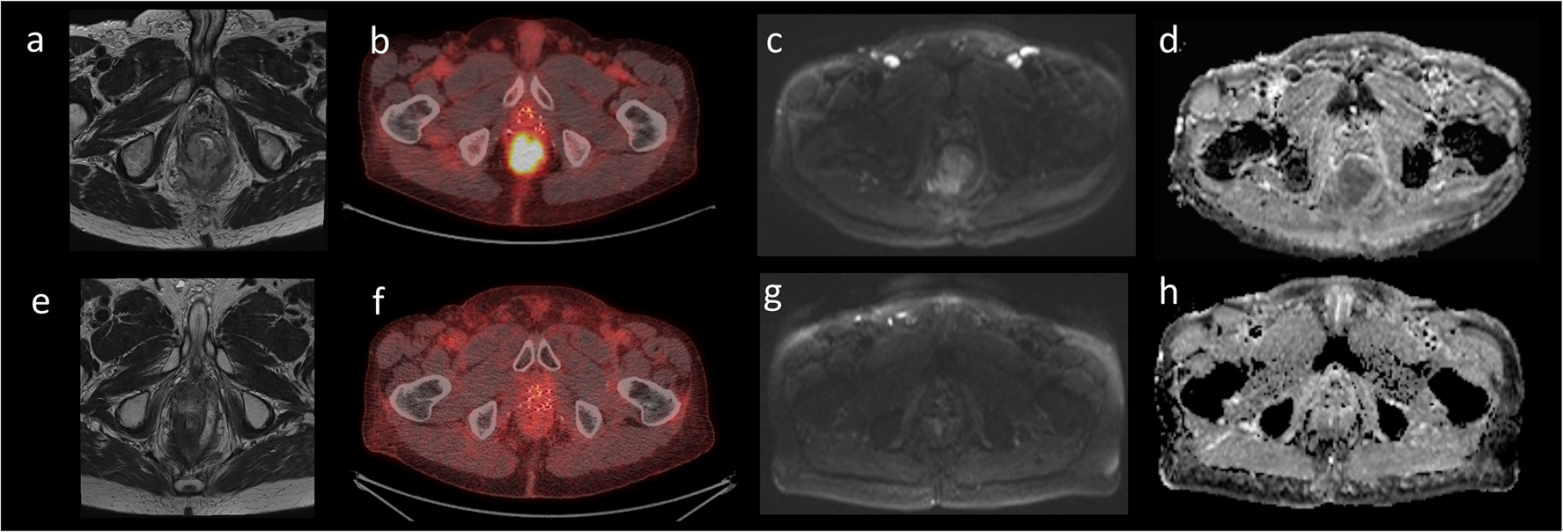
71-year-old male, brachytherapy seeds within the prostate. Axial images. MRI response assessment: partial, PET-CT response assessment: complete, combined response assessment: complete. a Pre-treatment T2W MRI demonstrates an almost annular locally advanced tumour centred at the anorectal junction extending into the perineum and rectum. b Pre-treatment PET-CT demonstrates a large metabolically active mass involving the anal canal and lower rectum; bilateral groin lymph nodes demonstrate moderate uptake and are likely involved. c DWI demonstrates diffusion restriction at the site of the tumour as well as inguinal node involvement (high signal). d The ADC confirms true restriction (low signal). e Post-treatment T2W MRI demonstrates reduced tumour volume which is largely fibrotic. Focal bowel wall oedema was present which may represent residual tumour or post-treatment inflammatory changes. No residual nodes are demonstrated. f Post-treatment PET-CT demonstrates no residual FDG avid disease at the site of the previous tumour and nodes. g The DWI sequence does not demonstrate restriction. h The lack of diffusion restriction is confirmed by the ADC map. Overall, due to the lack of residual FDG avid disease, the patient was considered as a complete responder. The focal bowel wall oedema on the response assessment MRI was most likely inflammatory in nature.
